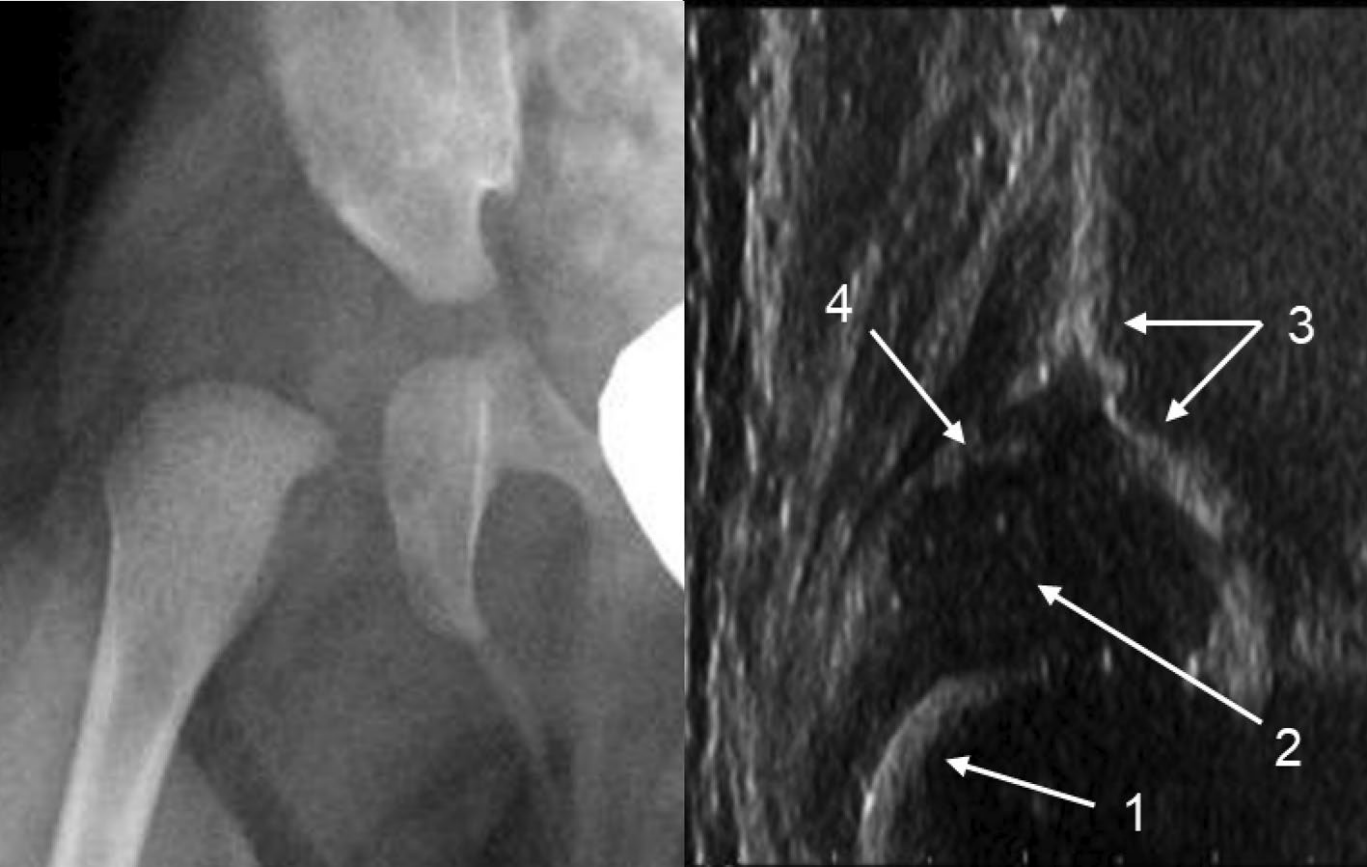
Hip anteroposterior radiography image (left) and hip ultrasonography image of the same hip (right). The patient was a 2-month-old girl. IHDI grade 2, Graf type D. Anatomical interpretation of the ultrasonography image: 1, bony part of the femoral neck. 2, cartilaginous femoral head. 3, bony part of acetabular roof. 4, acetabular labrum. *IDHI* International Hip Dysplasia Institute.
